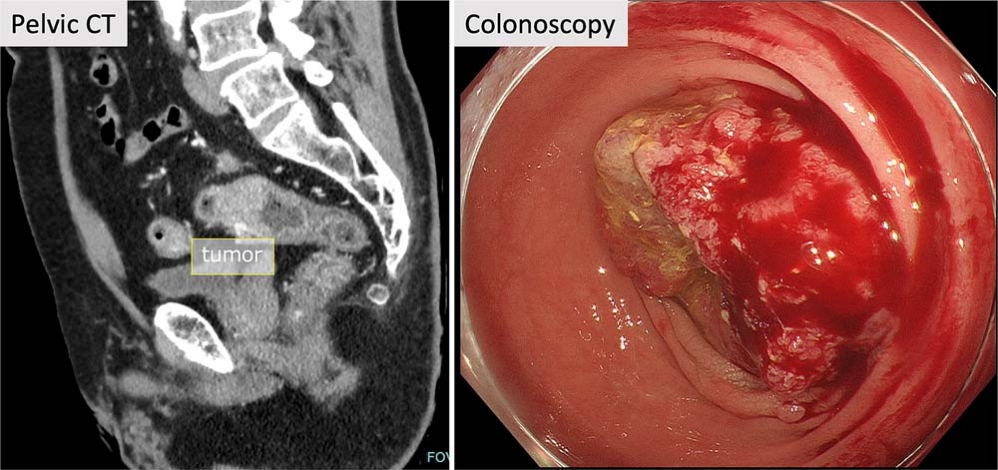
Pelvic CT and colonoscopy. Pelvic CT showed a tumor in the upper rectum, and rectal stenosis with the tumor was observed in colonoscopy.
